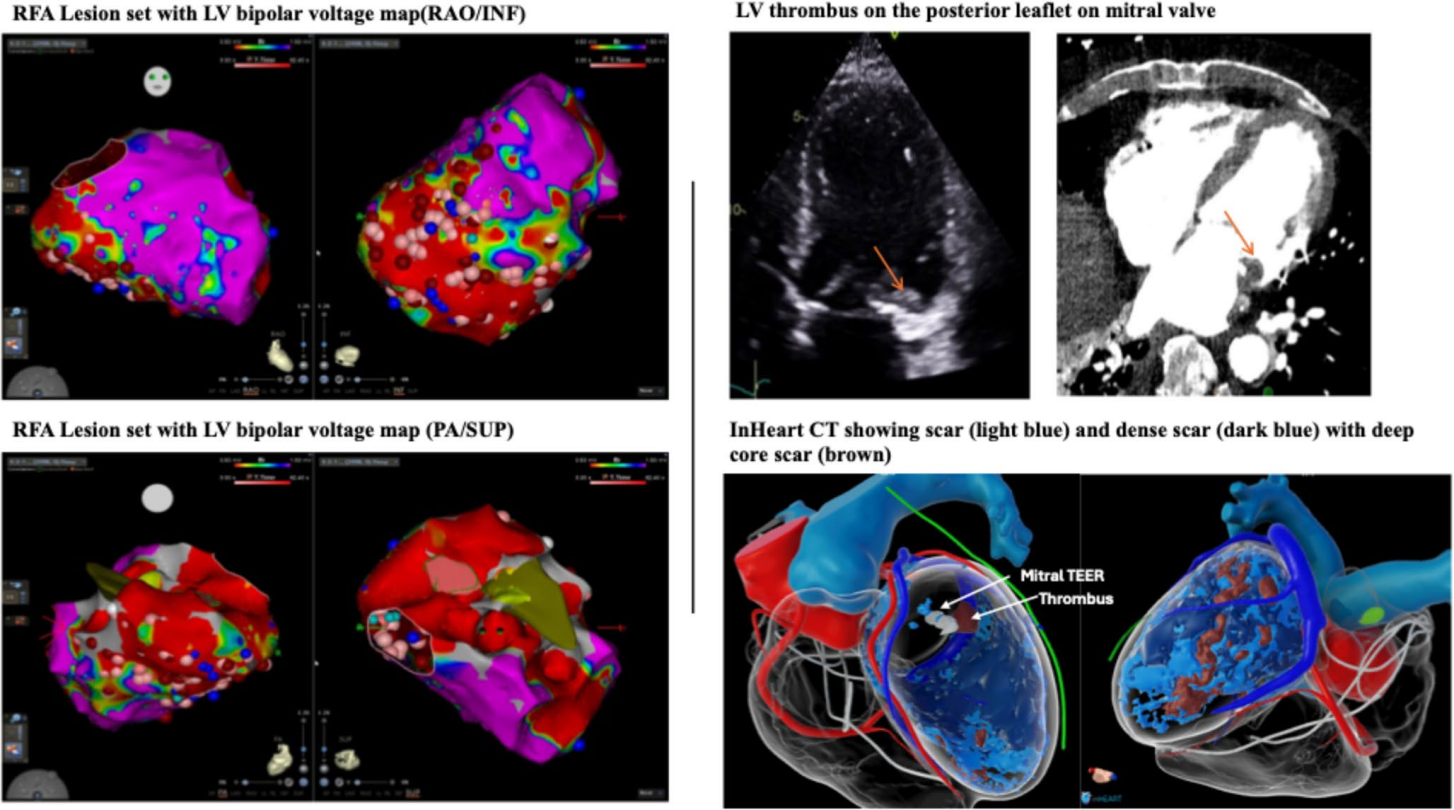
Electro-anatomical Mapping during VT ablation with echocardiogram and InHeart CT
